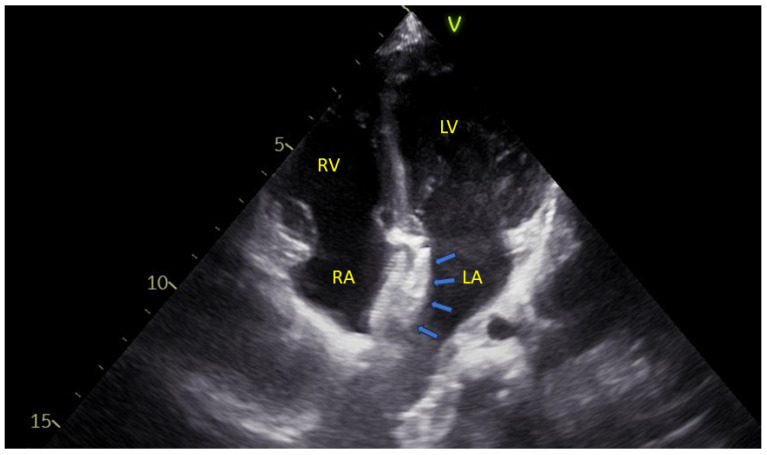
An apical four-chamber echocardiographic view showing the two disks of an atrial septal defect closure device (blue arrows) positioned within the interatrial septum. LA—Left Atrium; LV—Left Ventricle; RA—Right Atrium; RV—Right Ventricle.
